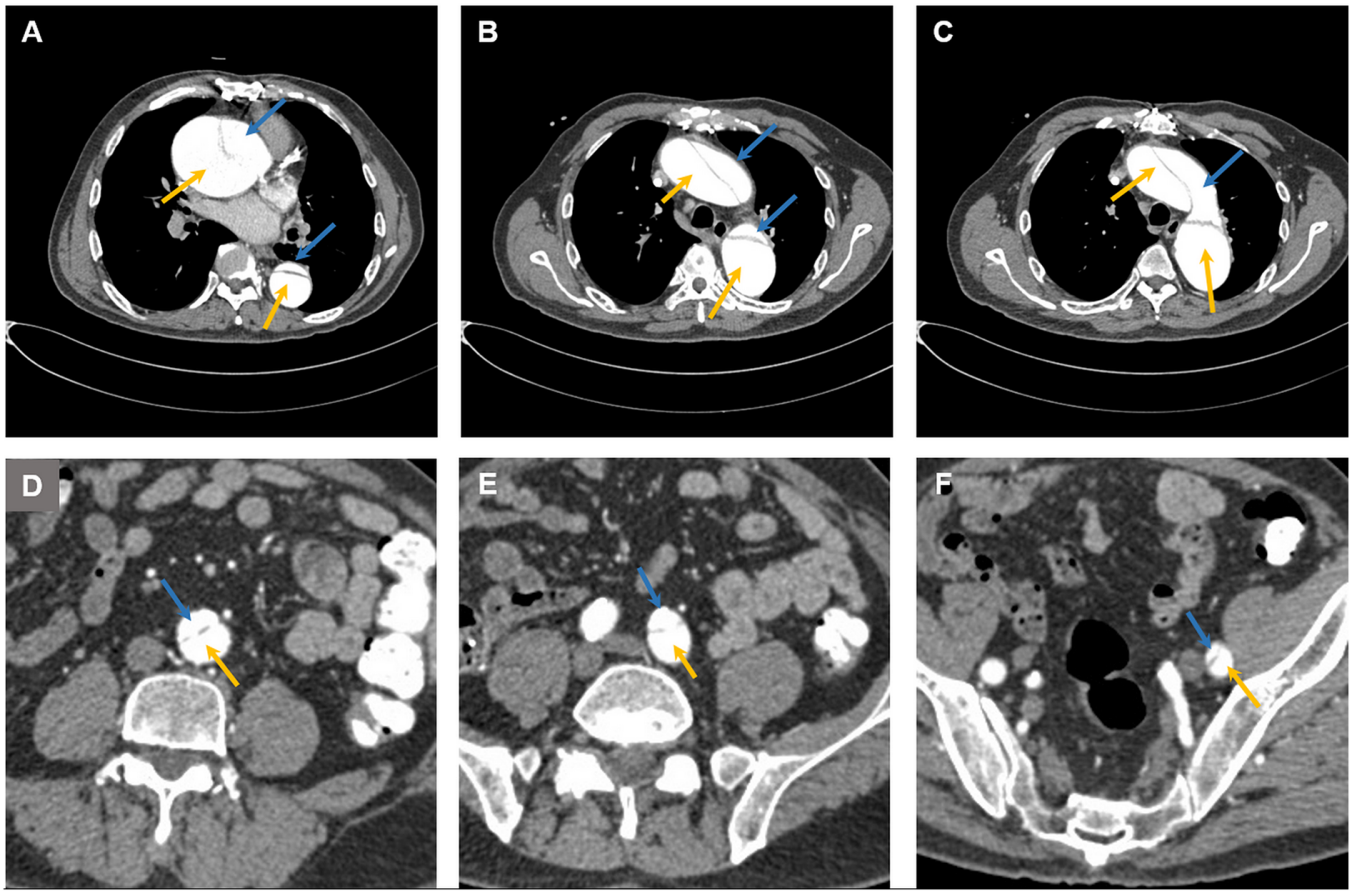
Computed tomography images showing aortic dissection at (A) the aortic root, (B) the ascending aorta, the descending aorta, (C) the aortic arch, (D) the abdominal aorta, (E) the left common iliac artery, and (F) the femoral artery. The blue arrow indicates the false lumen, and the yellow arrow indicates the true lumen.
